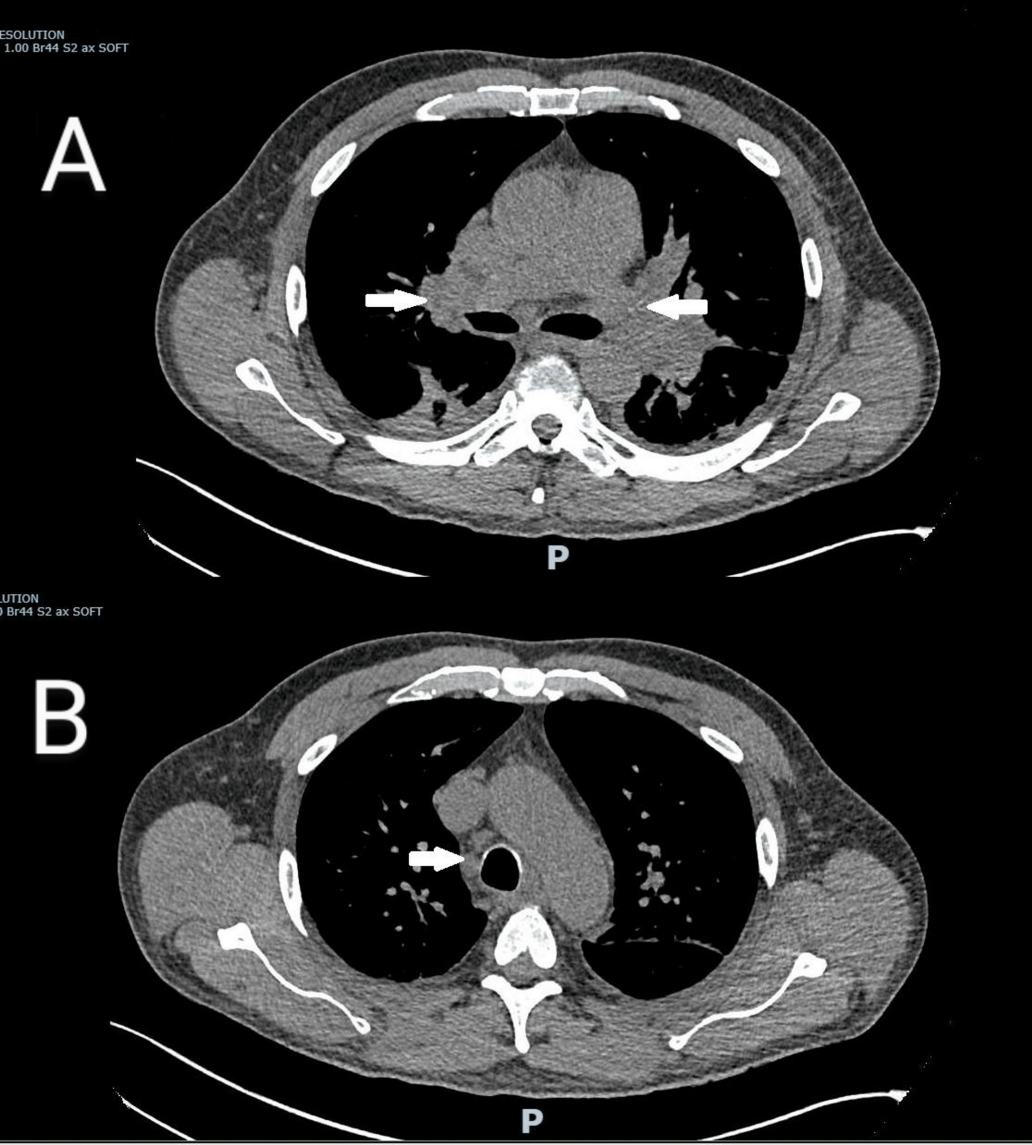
CT showing extensive bilateral hilar and mediastinal lymph nodes Note the arrows pointing to the enlarged lymph nodes in panels A and B
